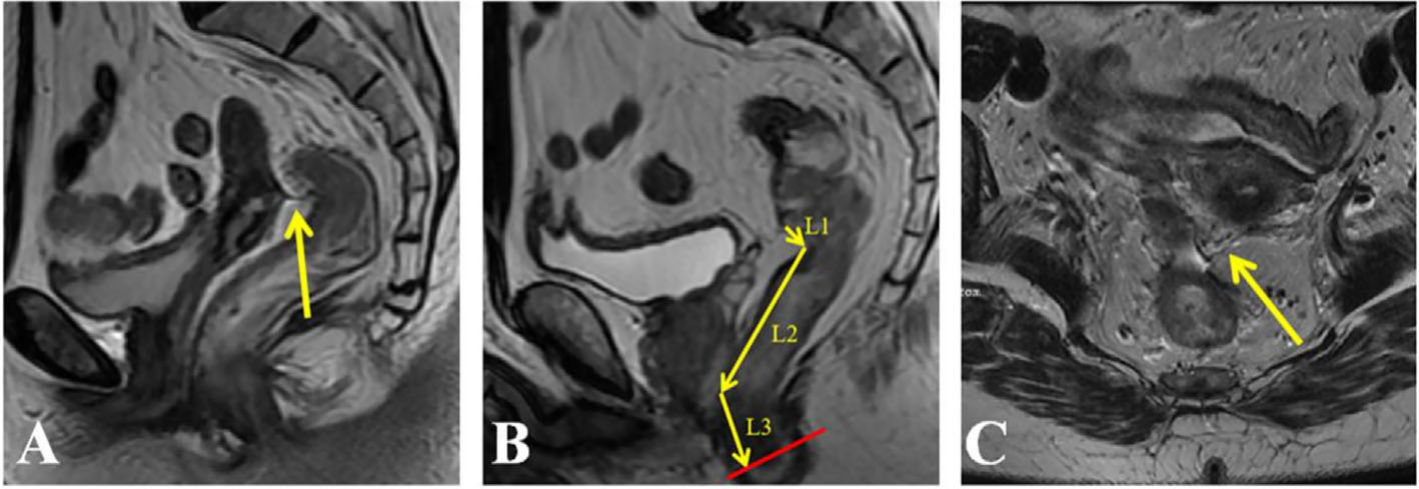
Identification of the anterior peritoneal reflection (APR) and measurement of tumor height on an MRI. (A) APR (arrow) in the sagittal plane; (B) the height of the tumor defined as the distance from the anal verge to the inferior tumor margin was measured in sagittal images; (C) APR (arrow) in the axial plane.
